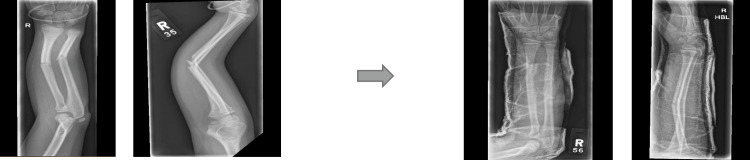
Radiograph 3 showing distal radius/ulna fracture in AP/Lat views and the subsequent manipulated views AP: Anteroposterior; Lat: Lateral
